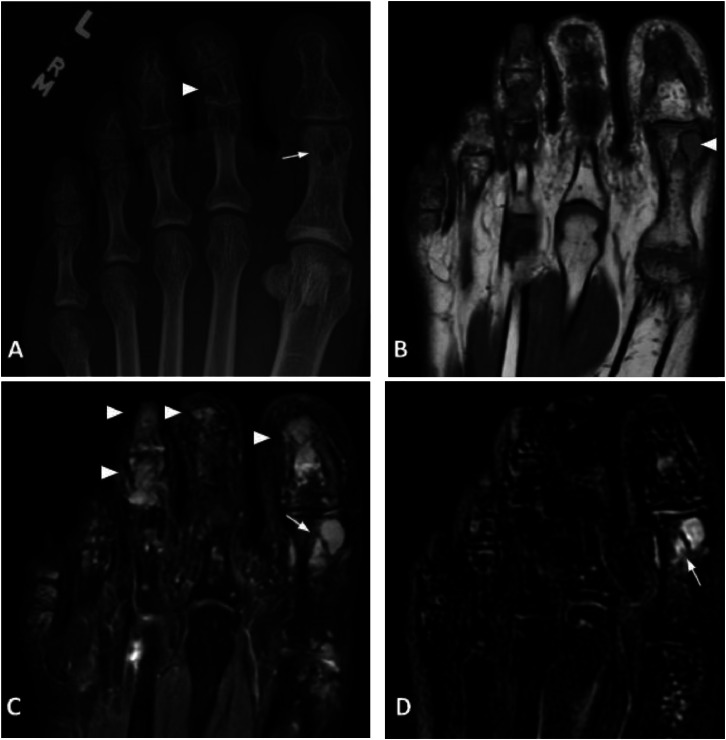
(A) AP Radiograph of the left foot demonstrates multifocal lucencies with erosive changes along the phalanges (white arrowhead). There is a prominent lucent lesion in the distal aspect of the proximal hallux (white arrow). (B) Axial T1 image of the left foot at the level of the first digit shows bilobed T1 hypointense lesion in the distal aspect of the first proximal phalanx (white arrow), corresponding with lucent lesion noted on prior radiograph. Additional T1 hypointense lesions (white arrowheads) are visualized in the second and third phalanges. (C) Axial STIR image of the left foot shows bilobed T2 hyperintense lesion in the first proximal phalanx (white arrow). Note additional areas of STIR hyperintensity in the first distal phalanx, middle and distal third phalanx, and distal fourth phalanx (white arrowheads). (D) Axial T1 postcontrast subtraction image of the left foot shows enhancement within the bilobed first proximal phalanx lesion (white arrow).
